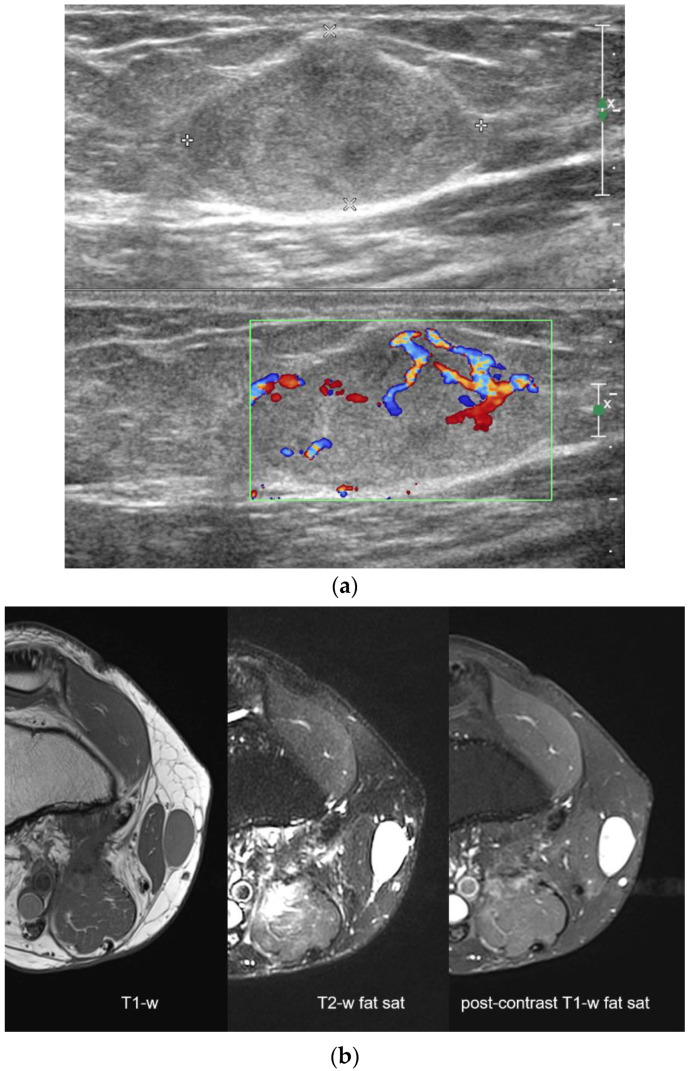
A 38-year-old man operated for a localized liposarcoma of the popliteal fossa. Images obtained after one year show a recurrence. Ultrasound images obtained at the medial aspect of the knee show a hyperechoic nodule (a) with intralesional color-Doppler signals and some slightly hypoechoic intralesional areas. MRI images (b) show a nodule isointense on T1-weighted sequences and hyperintense on both T2-weighted sequences with fat saturation and post-contrast T1-weighted sequences. Both US and MRI were reported as recurrence.
